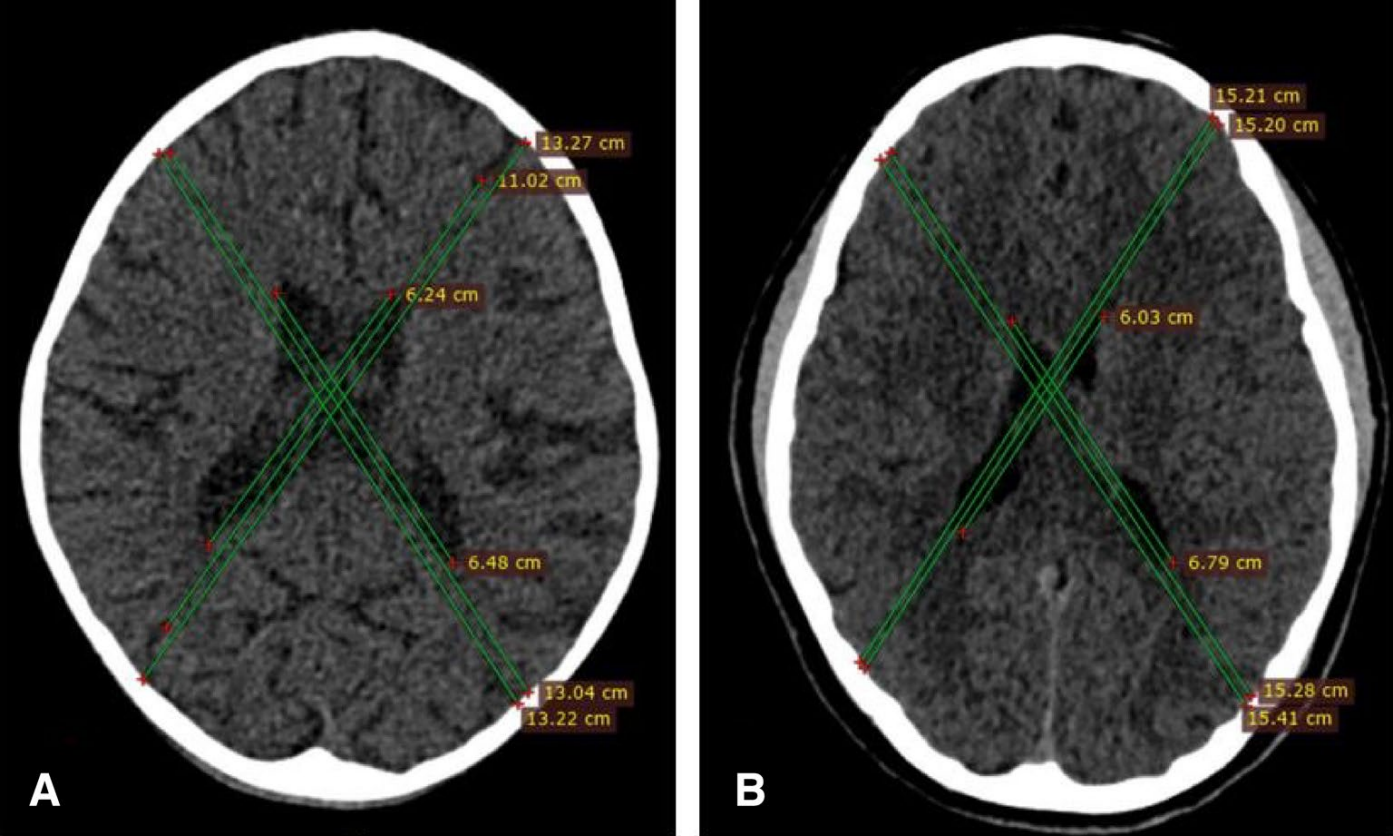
Brain CT scan sections at the widest part of the lateral ventricular body. (A) A case of brain atrophy from a 6-year-old male child showing prominent sulci and enlarged lateral ventricular body with a DBF value of 0.58 consistent with grade-II brain atrophy. (B) A normal brain volume of a 13-year-old male child showing effaced sulci intimately related to the calvarium, normal size lateral ventricular body with a DBF value of 0.81.
